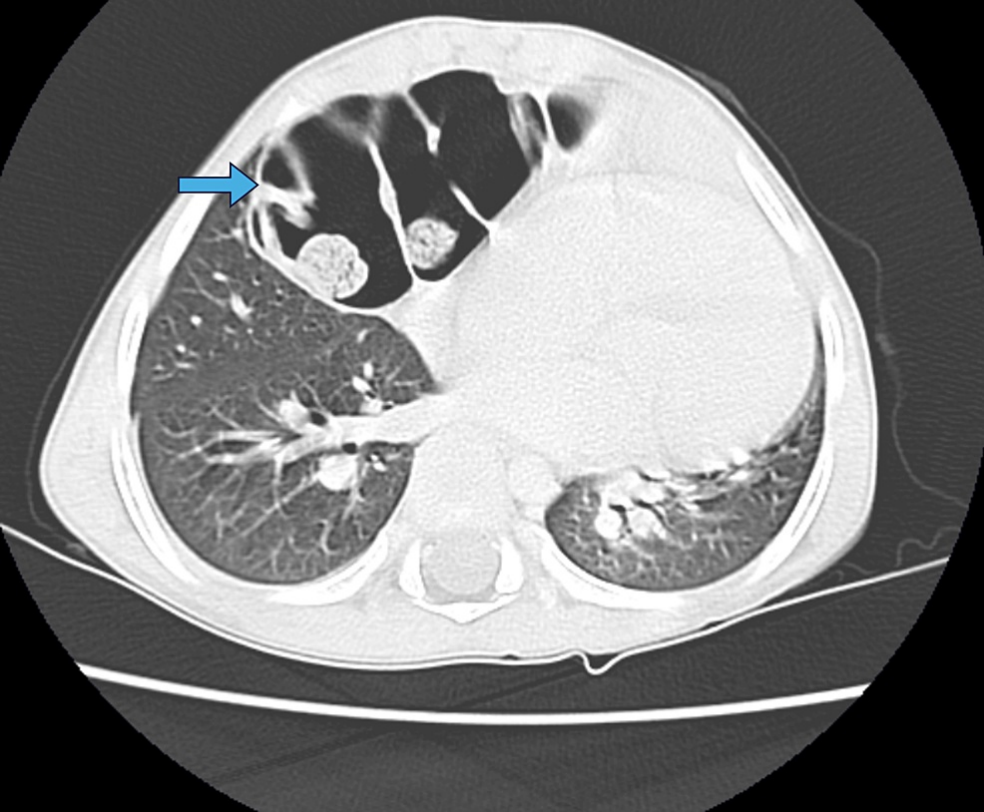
Axial section of the chest CT scan showing herniation of the colon into the thorax (blue arrow).
